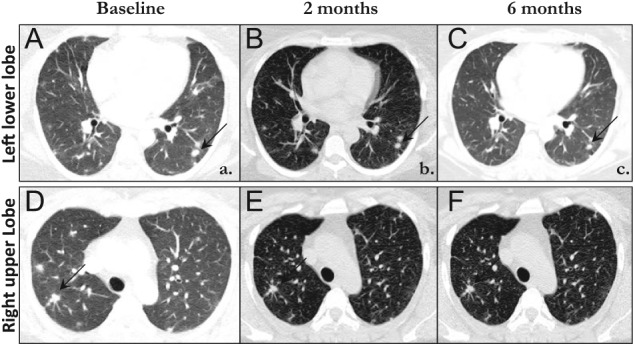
Computed tomography images of the chest are shown at baseline, 2, and 6 months after initiation of treatment with denosumab. Two representative lesions in the right upper lobe and left lower lobe are shown. The size of the shown lung nodules are smaller at 2 months of (B and E) treatment compared with baseline (A and D). No further shrinkage is seen after 4 additional months of treatment (C and F).
